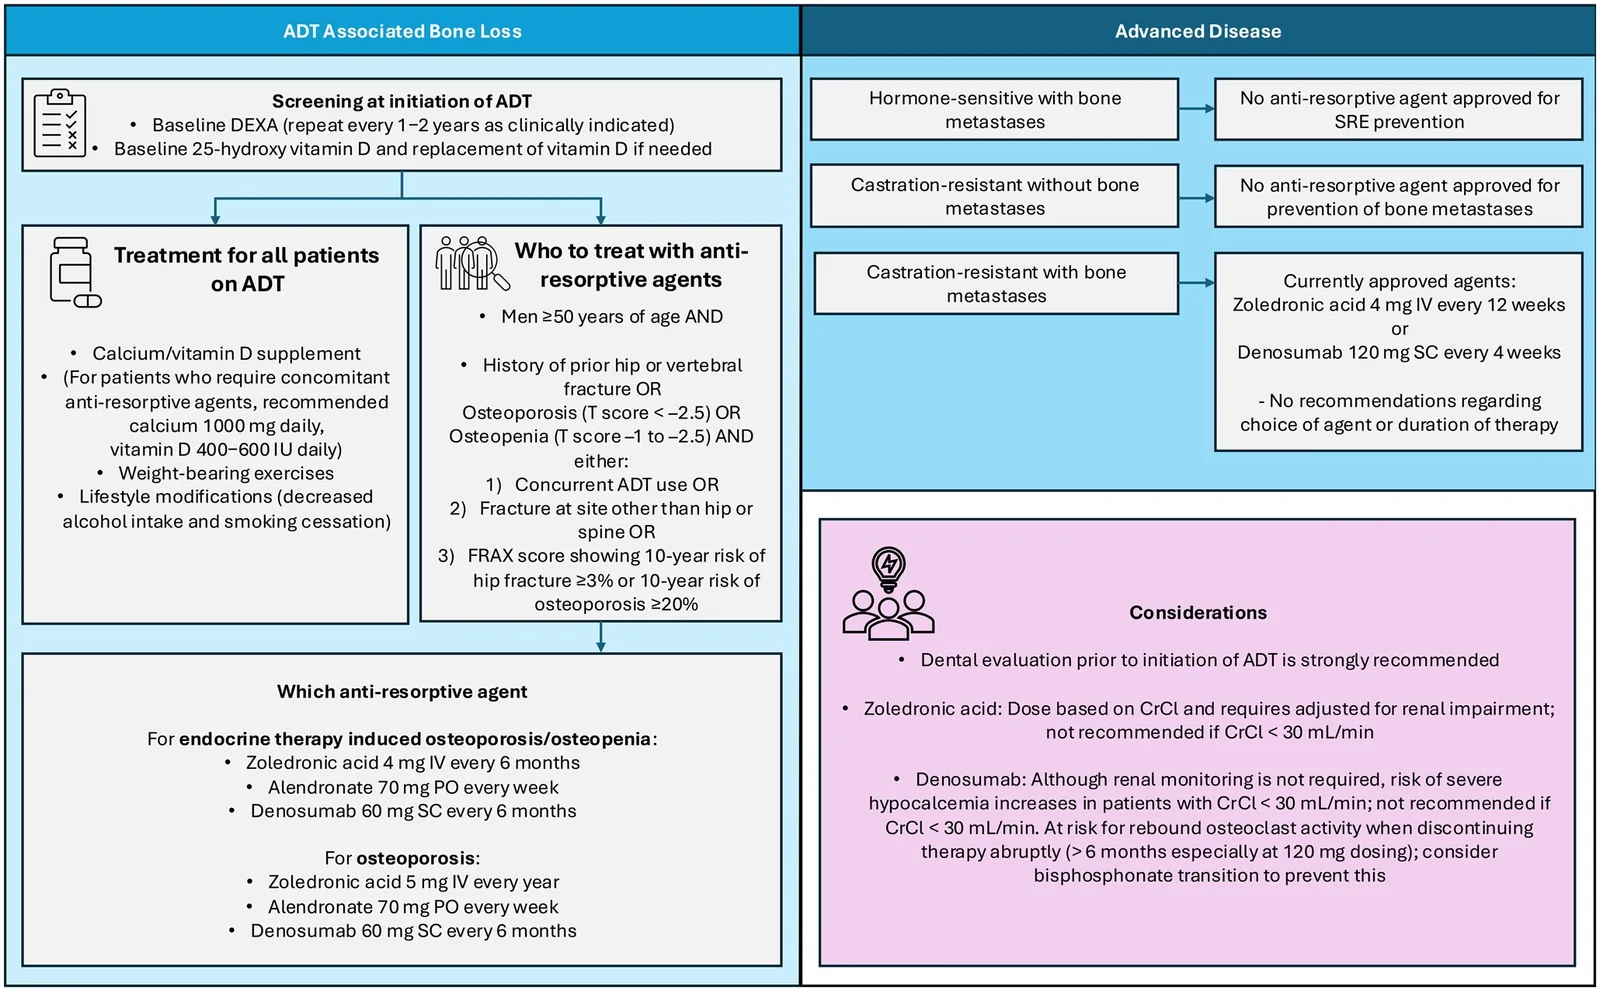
Management algorithm for bone health in prostate cancer. BHAs (zoledronic acid and denosumab) are not approved for use in mHSPC with bone metastases or CRPC without bone metastases. ADT, androgen-deprivation therapy; BHA, bone health agent; CrCl, creatine clearance; CRPC, castration-resistant prostate cancer; DEXA, dual-energy X-ray absorptiometry; FRAX, Fracture Risk Assessment Tool; IU, international units; IV, intravenous; mHSPC, metastatic hormone-sensitive prostate cancer; PO, orally; SC, subcutaneous; SRE, skeletal-related event.
